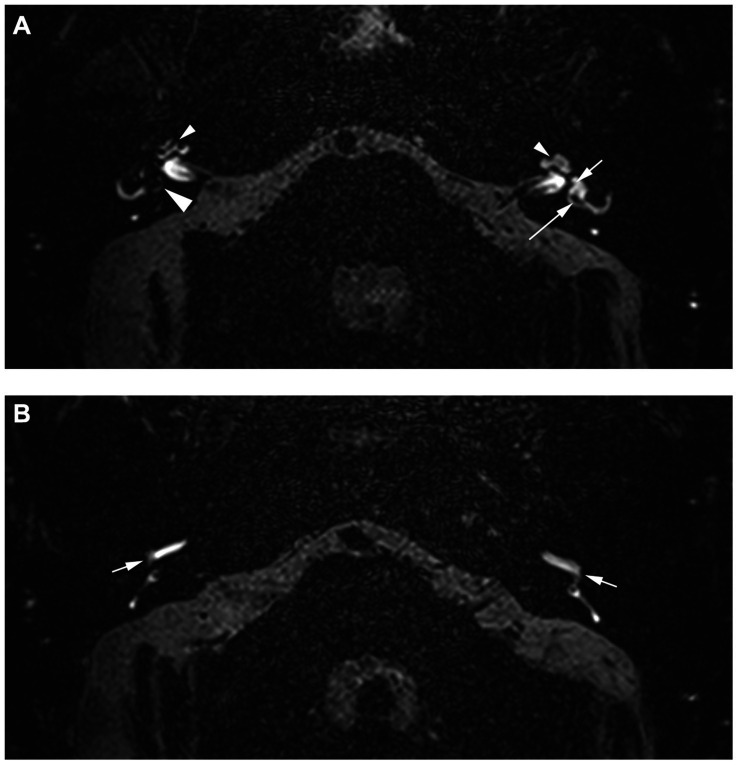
A 60-year-old man experiencing episodic attacks of vertigo with nausea and vomiting for the past 5 years. In addition, he also experiences tinnitus, a pressure sensation, and hearing loss on the right sight. Headaches and/or migraines are not reported. (A) An axial 4 h-delayed Gd-enhanced 3D SPACE FLAIR sequence at the level of the vestibule and the apical turn of the cochlea. There is a band-like area of signal loss in the apical turn of the cochlea on the right (small arrowhead), corresponding to complete obliteration of the scala vestibuli by the hydropic cochlear duct, consistent with a CEH grade II. This is in contrast to the normal slit-like appearance of the scala media on the left (small arrowhead). The vestibule on the right side is entirely filled up with the enlarged and fused saccule and utricle (large arrowhead), consistent with a VEH grade III. In contrast, the normal left side shows a separately visible saccule located anteriorly and medially (small arrow) and a posteriorly located utricle (large arrow). (B) An axial 4 h-delayed Gd-enhanced 3D SPACE FLAIR sequence at the level of the basal turn of the cochlea and the posterior semicircular canal. Increased PLE is observed in the basal turn of the cochlea on the right side, compared to the left (arrows). In conclusion, the increased PLE on the right, in combination with the grade II CEH and grade III VEH, is nearly pathognomonic for the diagnosis of (definite) MD.
